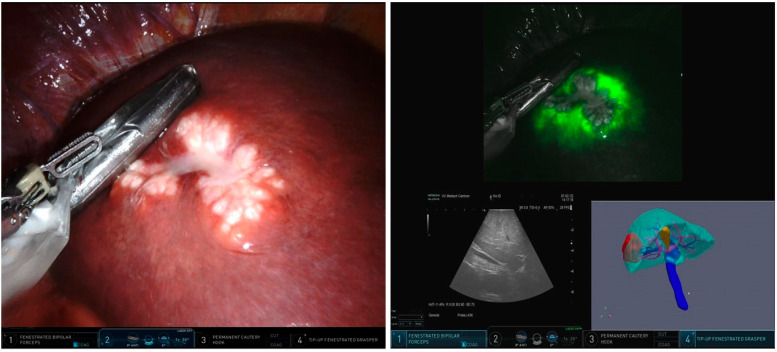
Surgeon’s perspective using the multimodality Robotic Liver Surgical Cockpit. (Left) image showing a white light image of liver and tumor in segment 6/7; (Right) image showing the near-infrared fluorescence overlay image of the tumor site in segment 6/7 in the upper panel; intraoperative ultrasonography of the tumor in the lower left panel and the Virtual Reality 3D model of the liver, tumor, and vital structures in the lower right panel.
